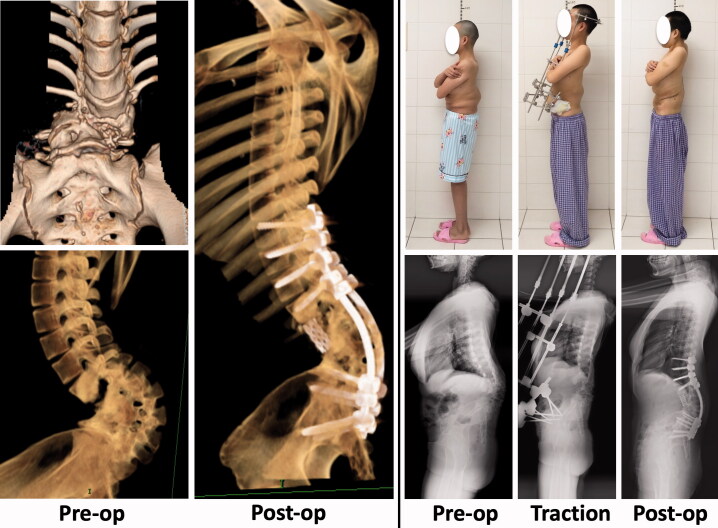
Case 3: CT revealed angular lumbar kyphosis, vertebral subluxation, absent vertebral bodies from L2 to L4 (presumably due to tuberculosis), and fusion of the L1–L5 laminas.
